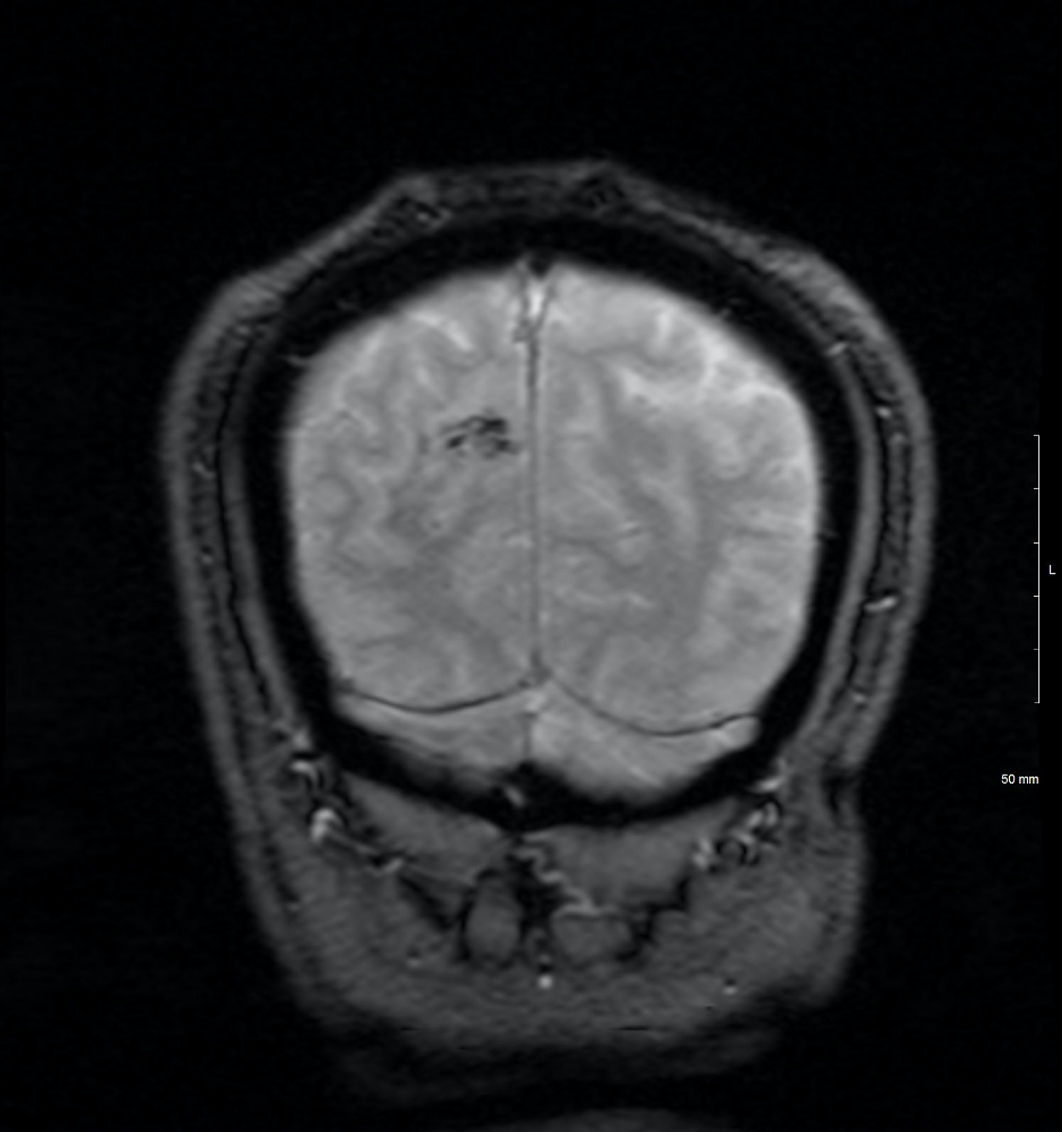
MRI brain, coronal view. T2 susceptibility weight images showing the evolution and decreased hemorrhage in the right posterior paramedian lobe.
